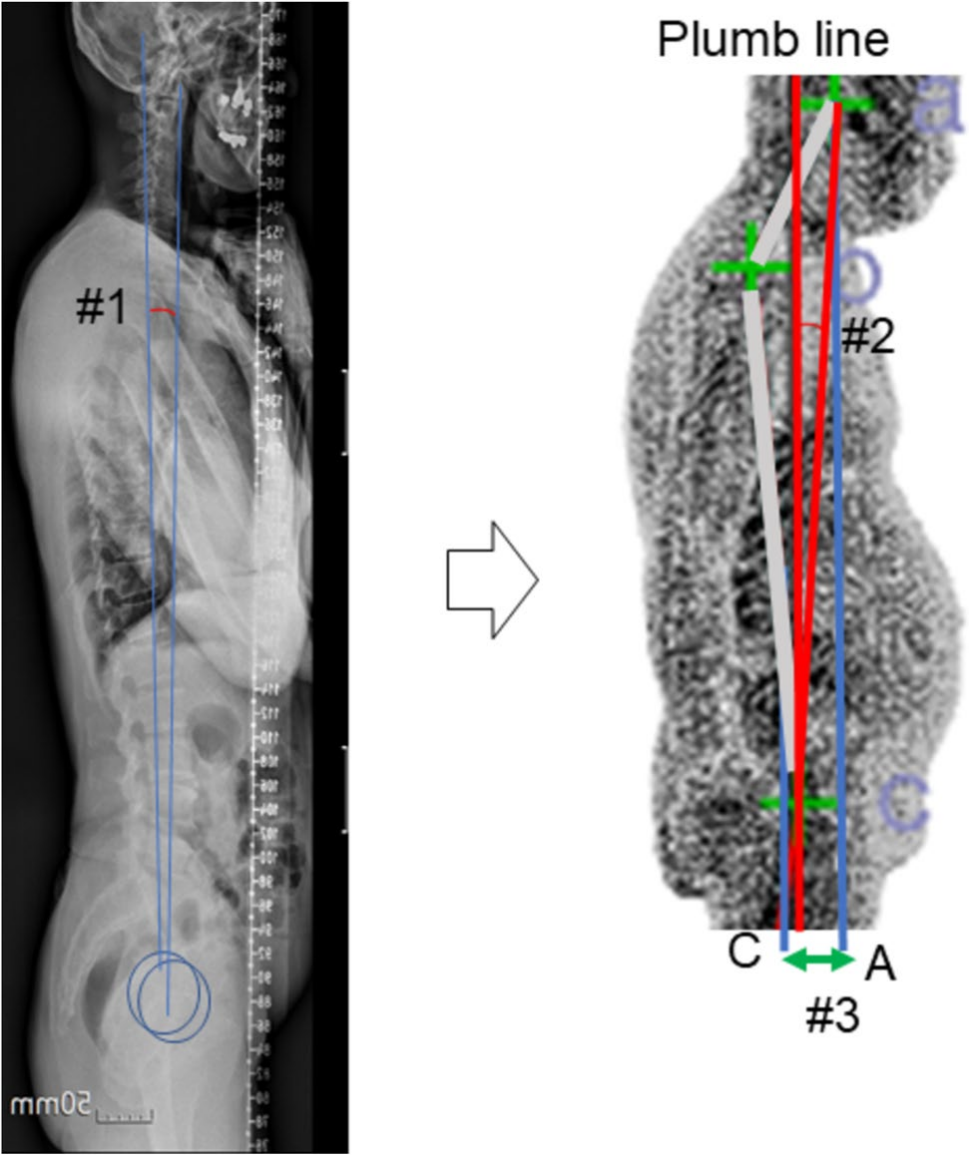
#1. ODHA on x-ray / #2. aAC_sag / #3. dAC_hor on the 3D scanner
